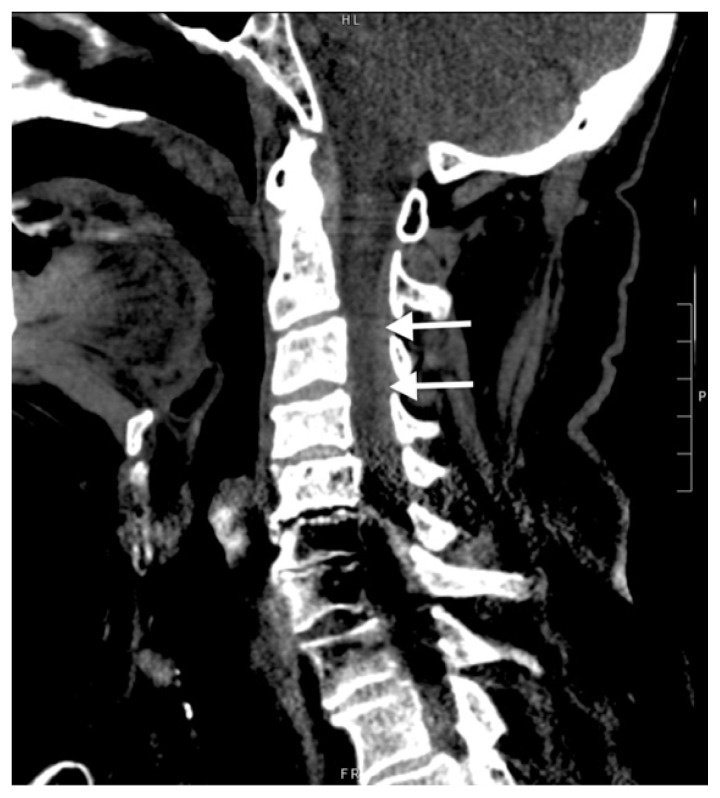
Sagittal non-contrast computed tomography of the cervical spine showing hyperdense dorsal epidural thickening (arrows) from second through seventh cervical vertebrae.
